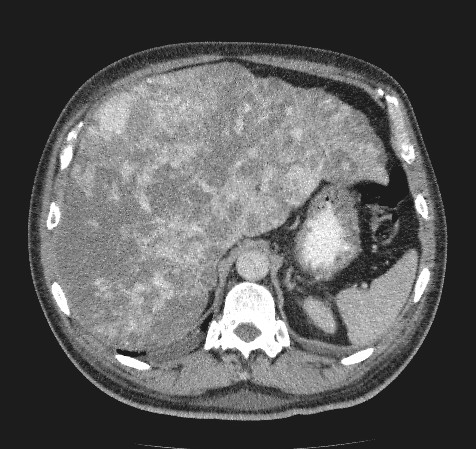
Abdominal CT scan showing complete replacement of liver parenchyma with liver tumour.
